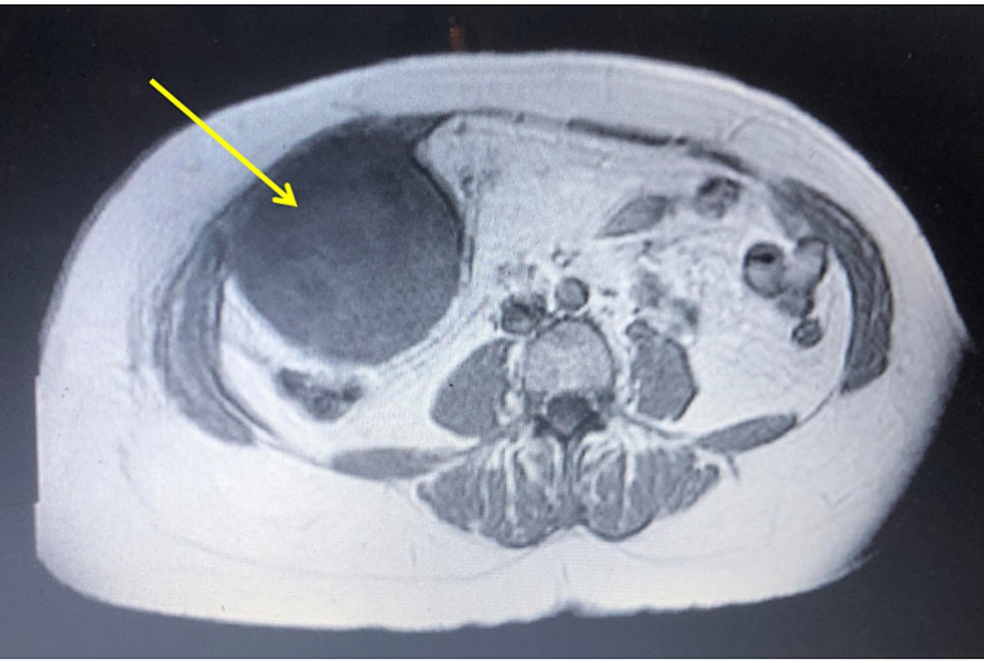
Magnetic resonance imaging abdomen with contrast enhancement demonstrating the ill-defined enhanced soft tissue tumour noted posterior to the right anterior abdominal wall (yellow arrow indicates tumour).
